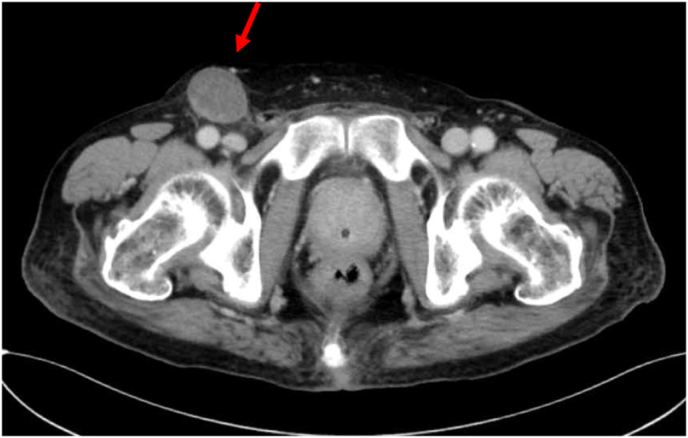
Contrast-enhanced computed tomography revealing an enlarged 3-cm right inguinal metastatic lymph node in penile cancer before lymphadenectomy (arrow).
